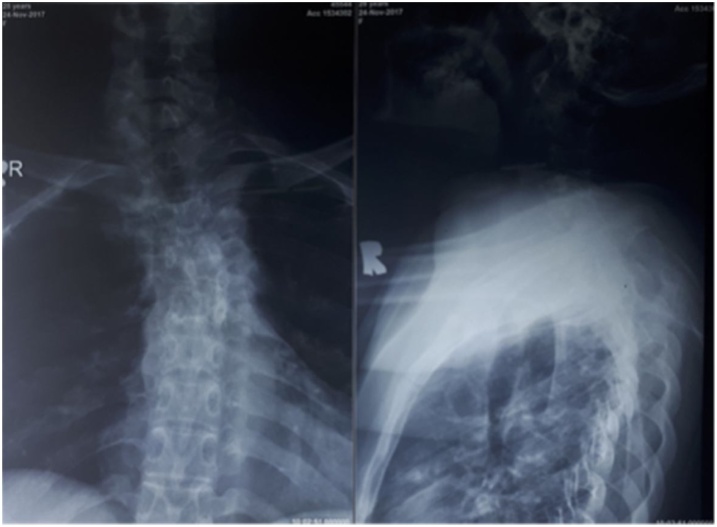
Preoperative radiograph of the patient.
